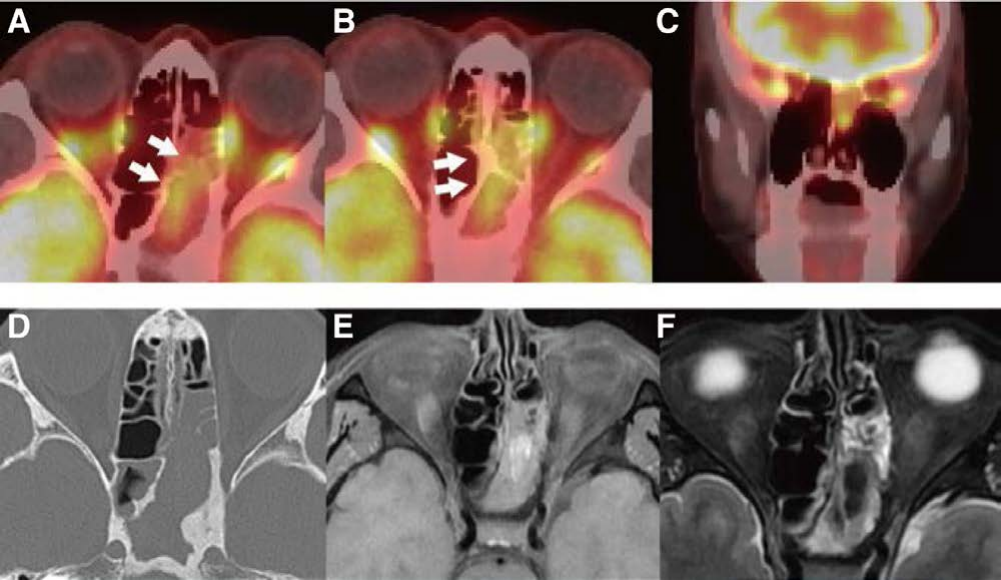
Preoperative imaging. (A–C) FDG uptake (SUVmax, 7.6) is detected in the left posterior ethmoidal and sphenoid sinuses (white arrow). (D) Axial CT scan shows bone destruction in the anterior wall of the sphenoid sinus and posterior nasal septum. In contrast, bone thickening is observed lateral to the posterior wall of the sphenoid sinus. (E and F) Mixed high-intensity and iso-intensity signal areas are observed in the lesion on T1-weighted imaging, and mixed iso-intensity and low-intensity signal areas are observed in the lesion on T2-weighted MRI scans. CT = Computed tomography, FDG = 18F-fluorodeoxyglucose, MRI = magnetic resonance imaging, SUVmax = maximum standardized uptake value.
